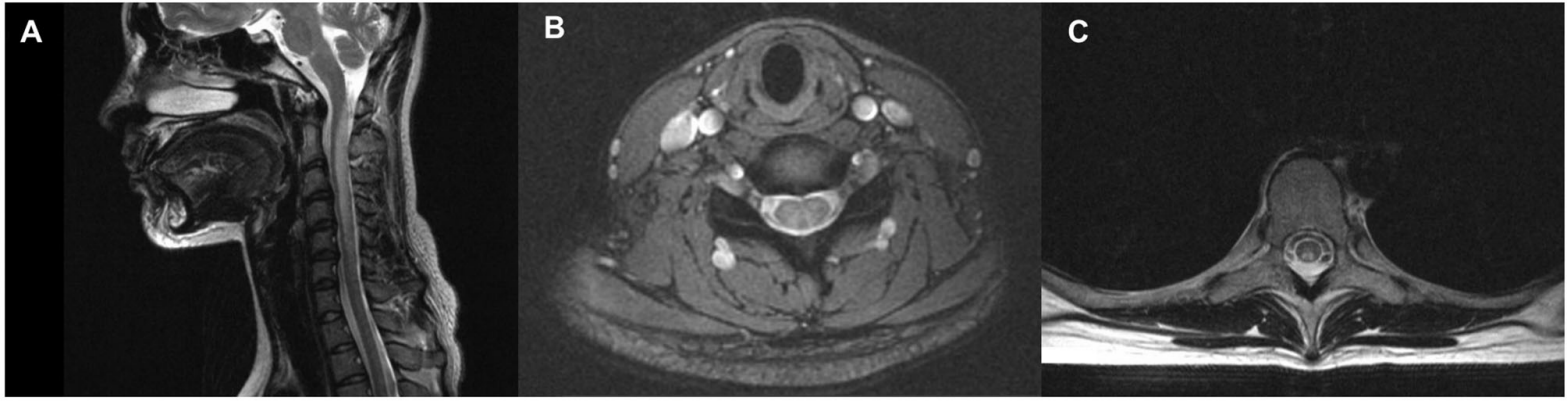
Spinal cord Magnetic Resonance Imaging (MRI) of a patient with N_2_O -abuse who complained of progressive unsteadiness while walking and limb numbness for 14 days. (A) Sagittal T2 MRI of the cervical spine showed a longitudinal hyperintensity signal C1–C6. (B) Axial gadolinium-enhanced T2 MRI showed symmetric and inverted V-shaped hyperintense signals in the dorsal columns of the cervical spinal cord. (C) Axial gadolinium-enhanced T2 MRI showed symmetric and inverted V-shaped hyperintense signals in the dorsal columns of the thoracic spinal cord.
